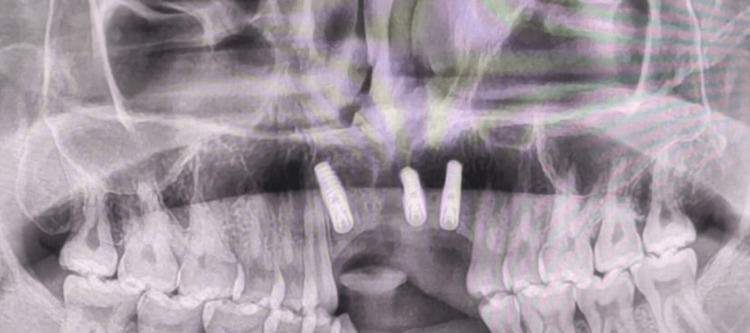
Postoperative radiograph after implant placement
